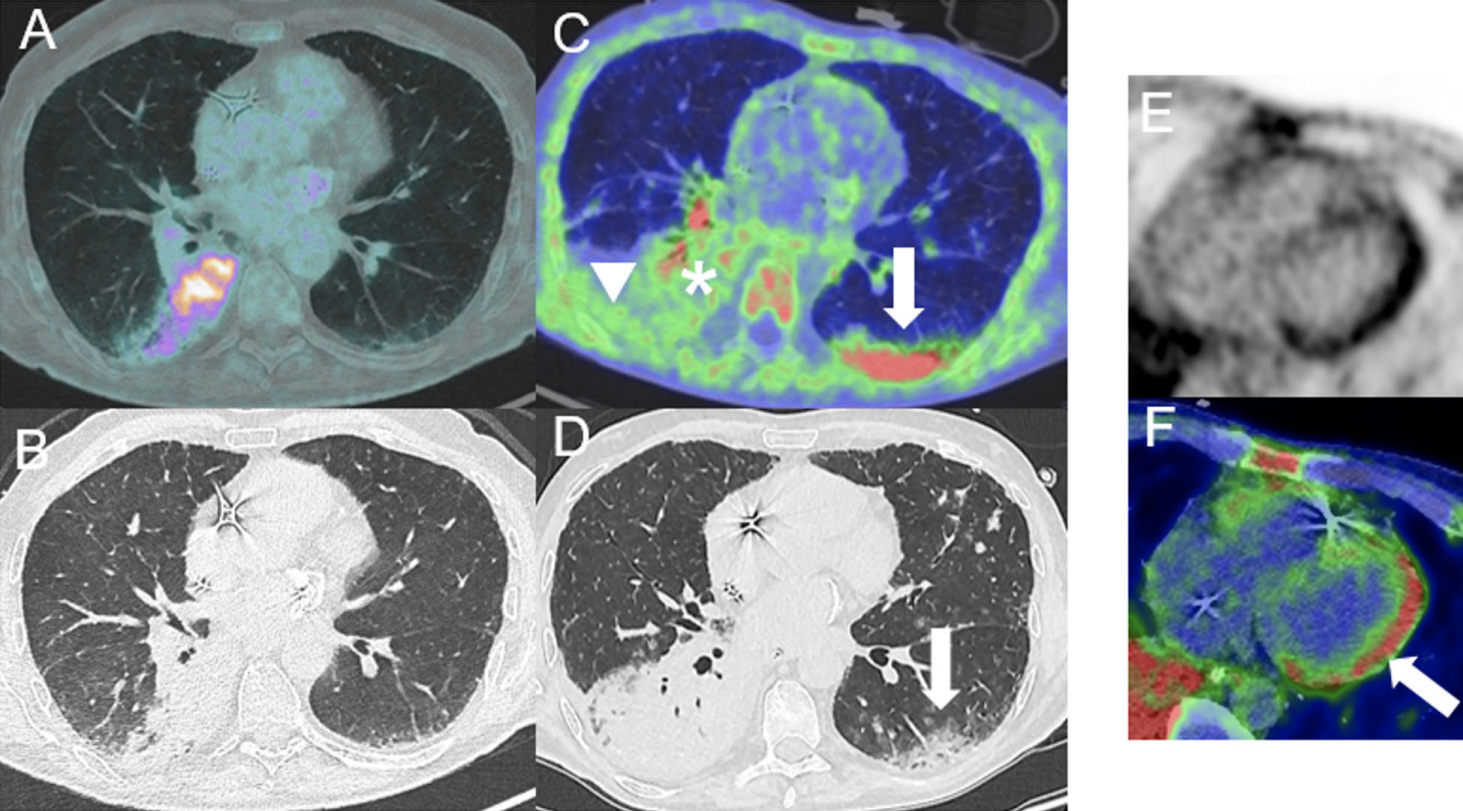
Immune-related myocarditis in a 61-year-old male with mucinous lung adenocarcinoma in right lower lobe [cT4 (>7cm) cN0 cM0] initially treated with carboplatin, vinorelbine and radiation therapy followed by consolidation treatment with durvalumab (anti-PD-L1). Baseline (before ICI) fused axial ^18^FDG PET/CT image (A) and corresponding axial CT image (B). After 2 cycles of durvalumab, the patient experienced severe dyspnea, atrial fibrillation leading to cardiogenic shock with clinical suspicion of ICI-related myocarditis. ^68^Ga-DOTATOC-PET/CT showed necrotic areas in the lung cancer [asterisk, (C)] with presence of peripheral inflammatory/infectious uptake [arrowhead, (C)] and newly appeared subpleural alveolar consolidations in the left lower lobe compatible with an organizing pneumonia [arrow, (C, D)]. ^68^Ga-DOTATOC-PET/CT showed diffuse myocardial uptake in the left ventricle (LV) [(E, F)], with an increased uptake ratio of 2.6 (SUVpeak LV_myocardium_/SUVmean LV_cavity_) suggestive of myocarditis [arrow, fused axial PET/CT image (F)].
